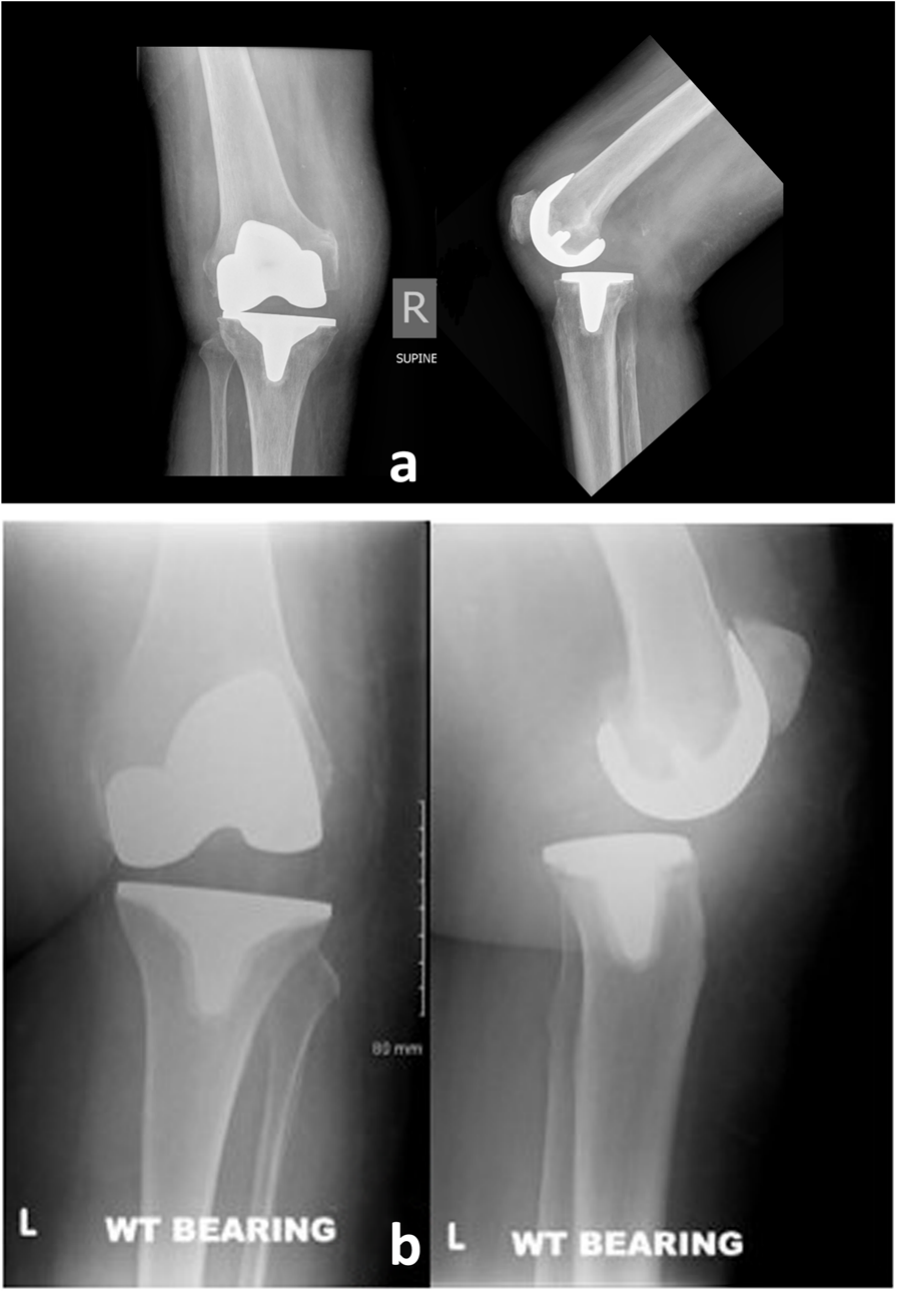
a X-ray showing an example of a posterolateral polyethylene spinout; there is posterior translation of the tibia on the lateral X-ray and on the anteroposterior (AP) view the lateral side of the joint space is closed down. b X-ray showing an example of a posteromedial polyethylene spinout; there is also posterior translation of the tibia on the lateral X-ray but on the AP view the medial side of the joint space is closed down
